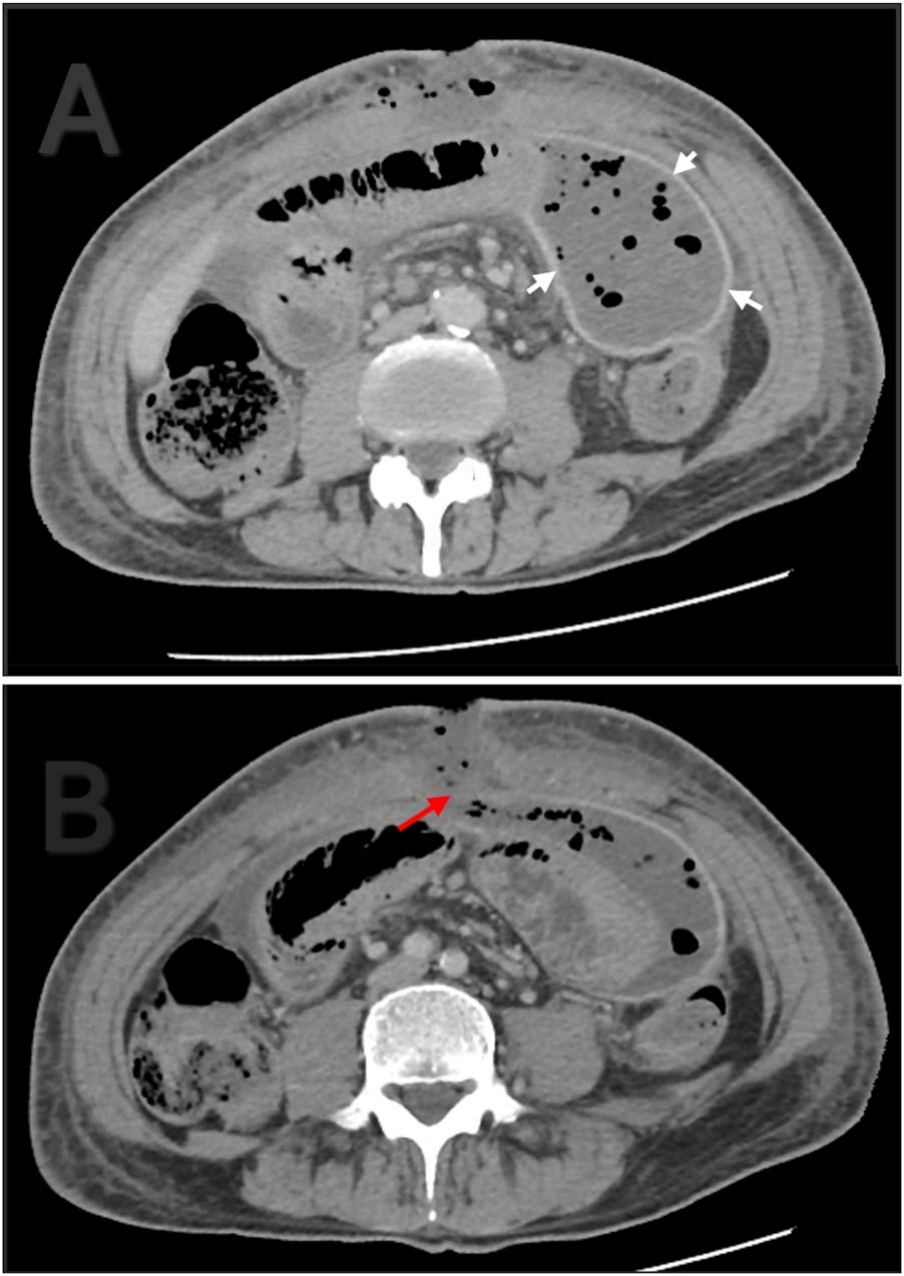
Contrast enhanced abdominal CT. (A) Thick and well localized capsule surrounding the collection (white arrows). (B) The collection is communicating with the atmosphere through a fascial dehiscence (red arrow).
